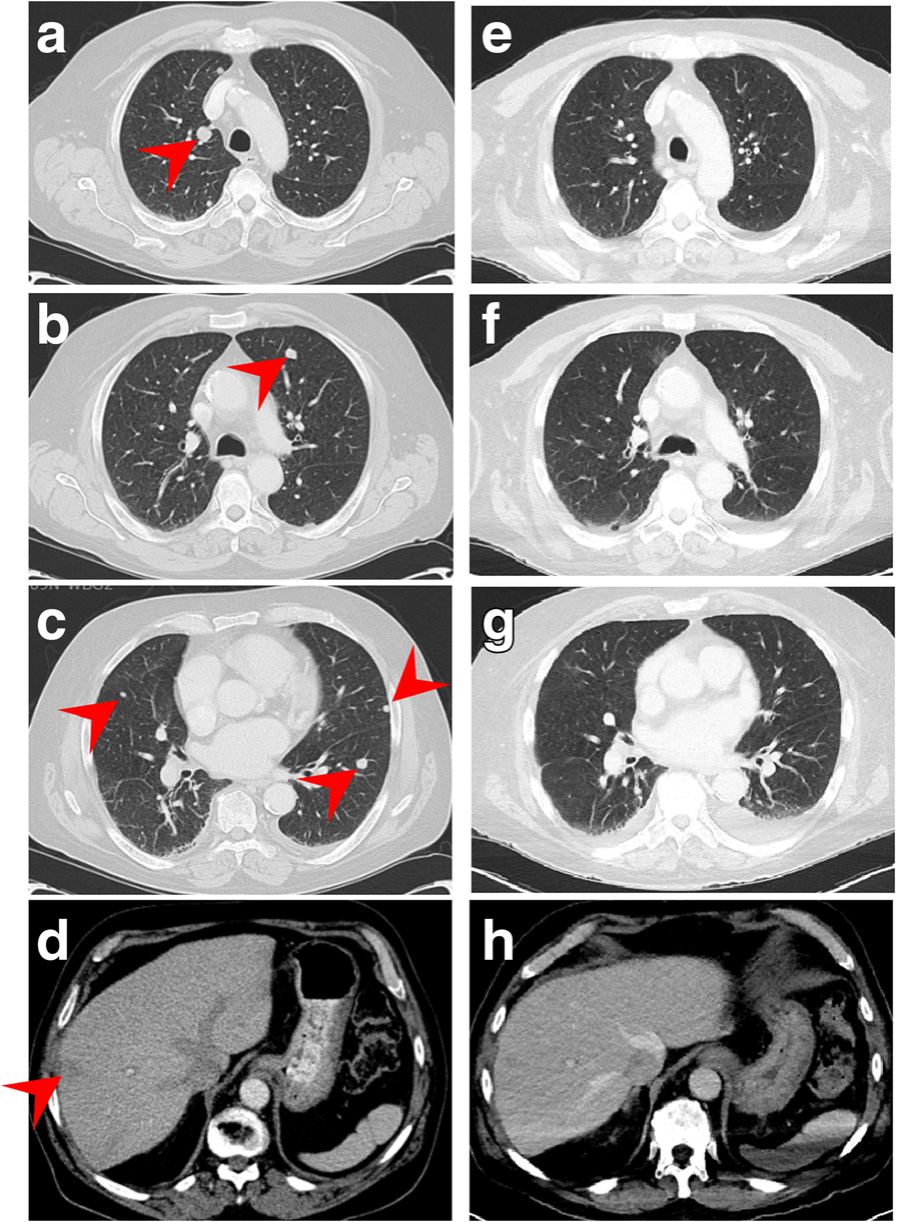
CT scans of the chest and abdomen show numerous bilateral pulmonary nodules measuring up to 43 mm (a-c) and liver lesions with a prominent 20 mm lesion (d) prior to immunotherapy initiation. All lesions are markedly improved (e-h) by four months after treatment with ipilimumab and nivolumab has started
